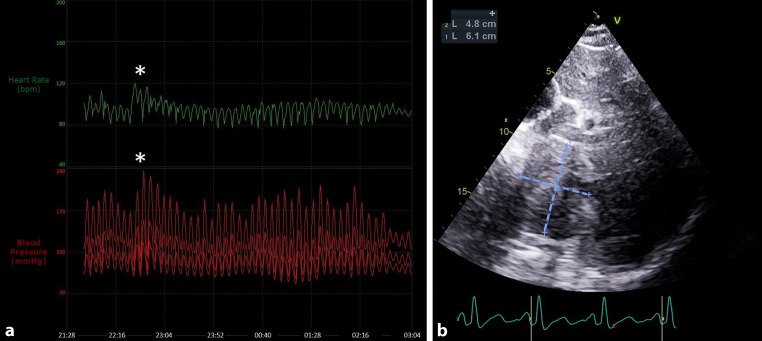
a Heart rate and invasive blood pressure monitoring documenting paroxysms of tachycardia and arterial hypertension over a period of approximately 5 h (*asterisk* denotes highest recorded value of 120 bpm for heart rate and 240/120 mm Hg for blood pressure). b Echocardiographic subcostal view showing a heterogeneous mass with well-defined borders (size: ~48 × 61 mm) under the liver
